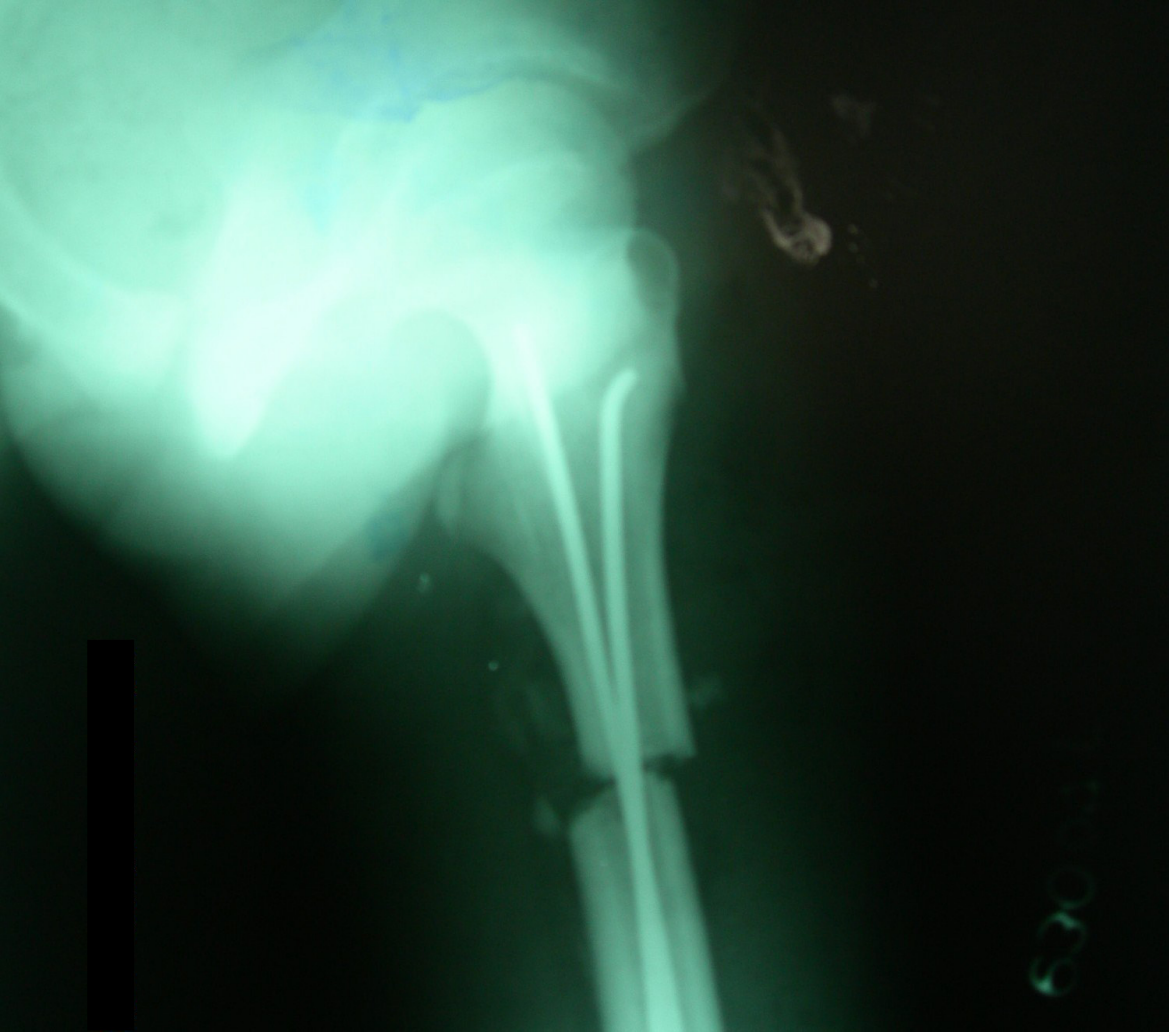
Lateral radiographs of a patient showing good nail position in proximal femur on immediate postoperative radiographs
